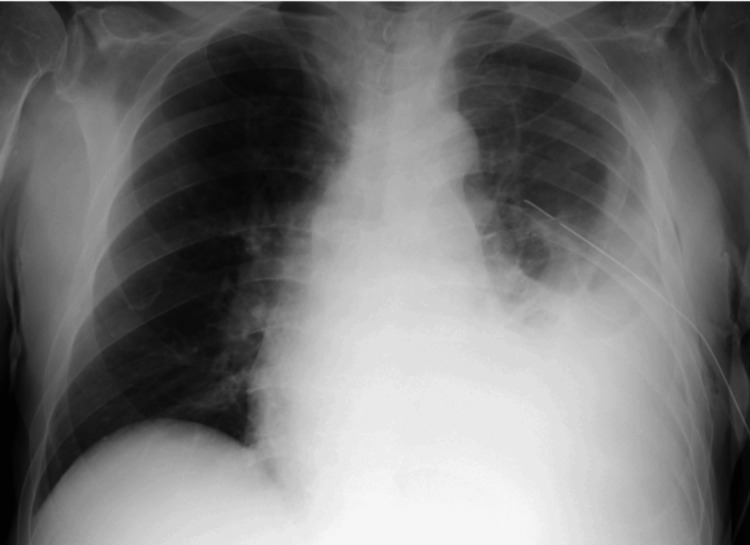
Chest radiograph (orthostatic position) showing the chest drain in the left lung field.
